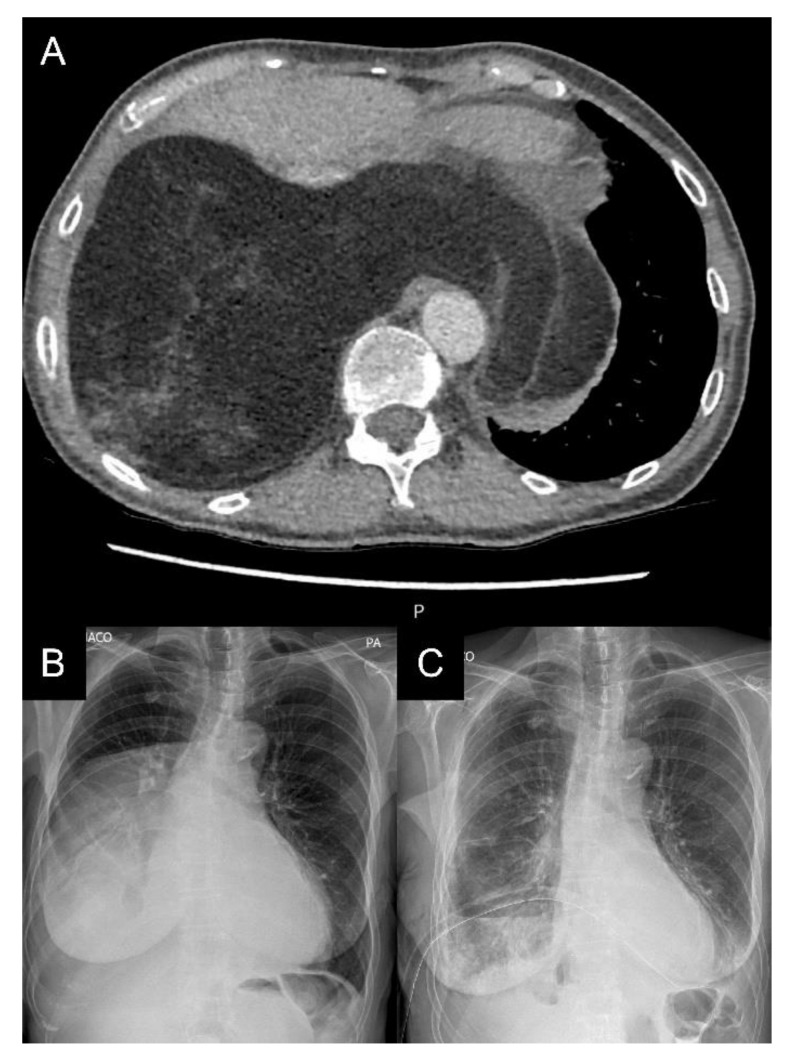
Radiological imaging of the patient during the first surgery. (A): CT with a massive lesion in the right thoracic cavity, 15.1 cm × 22.9 cm × 21.9 cm in size. (B,C): Pre- and postoperative chest X-rays of the patient.
